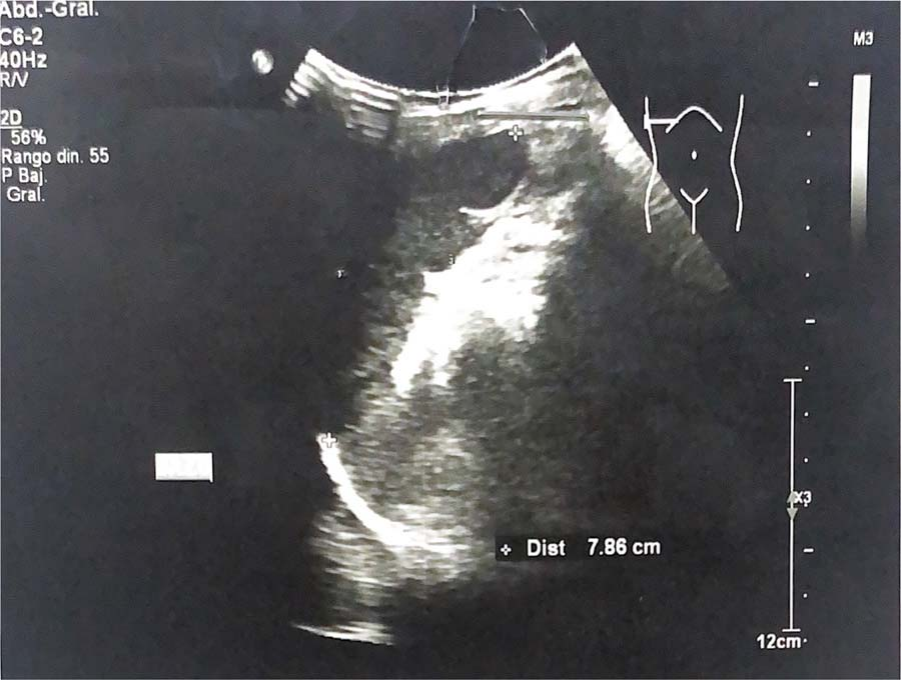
Abdominal ultrasound. Ultrasound image on left upper quadrant showing left sided liver.
